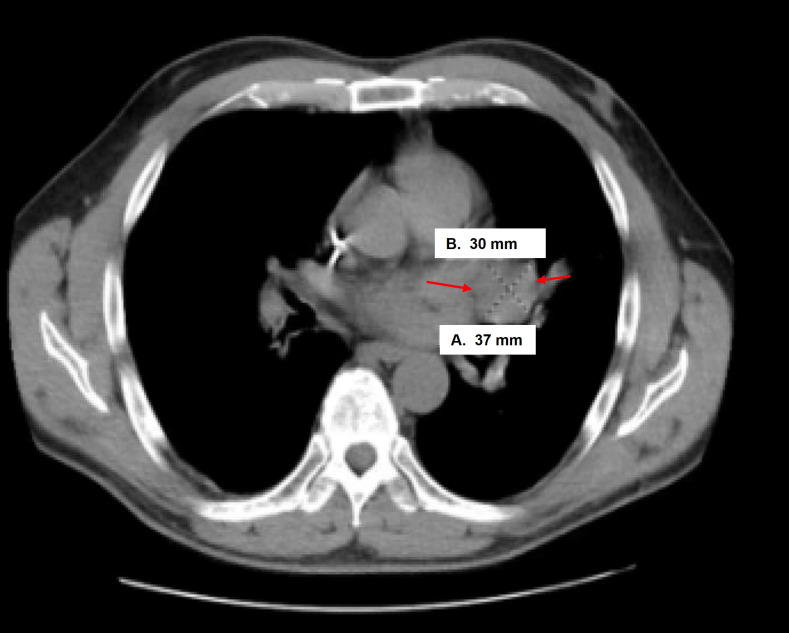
Computed tomography (CT) scan findings of clinical case 2 with enlarging hilar mass at 30 mm × 37 mm size (arrows)
